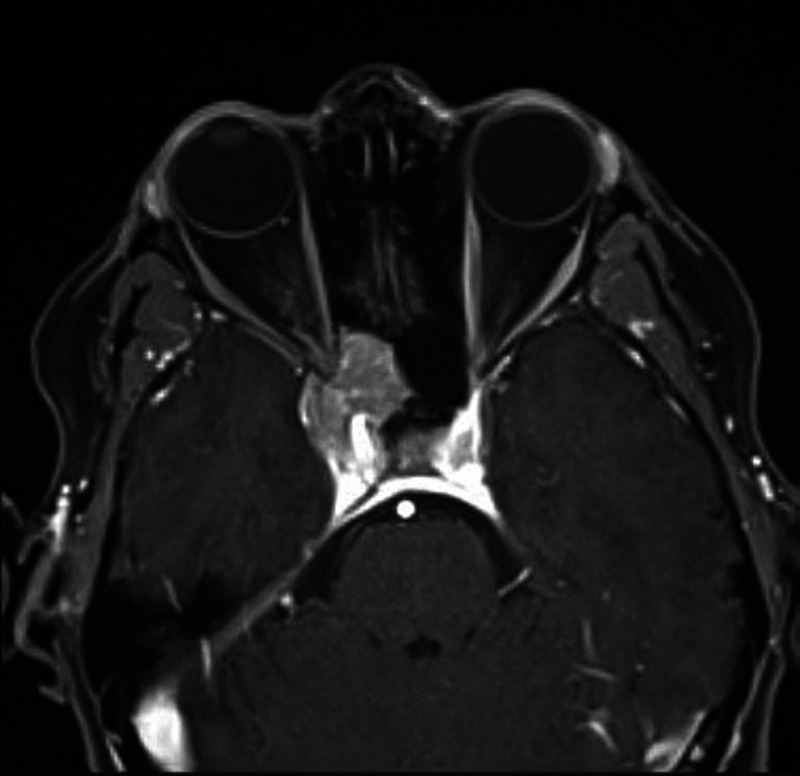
Axial post-contrast T1-weighted MRI showing an enhancing lesion in the right cavernous sinus, later pathologically proven to represent a plasmacytoma. T1, longitudinal relaxation time; MRI, magnetic resonance imaging
